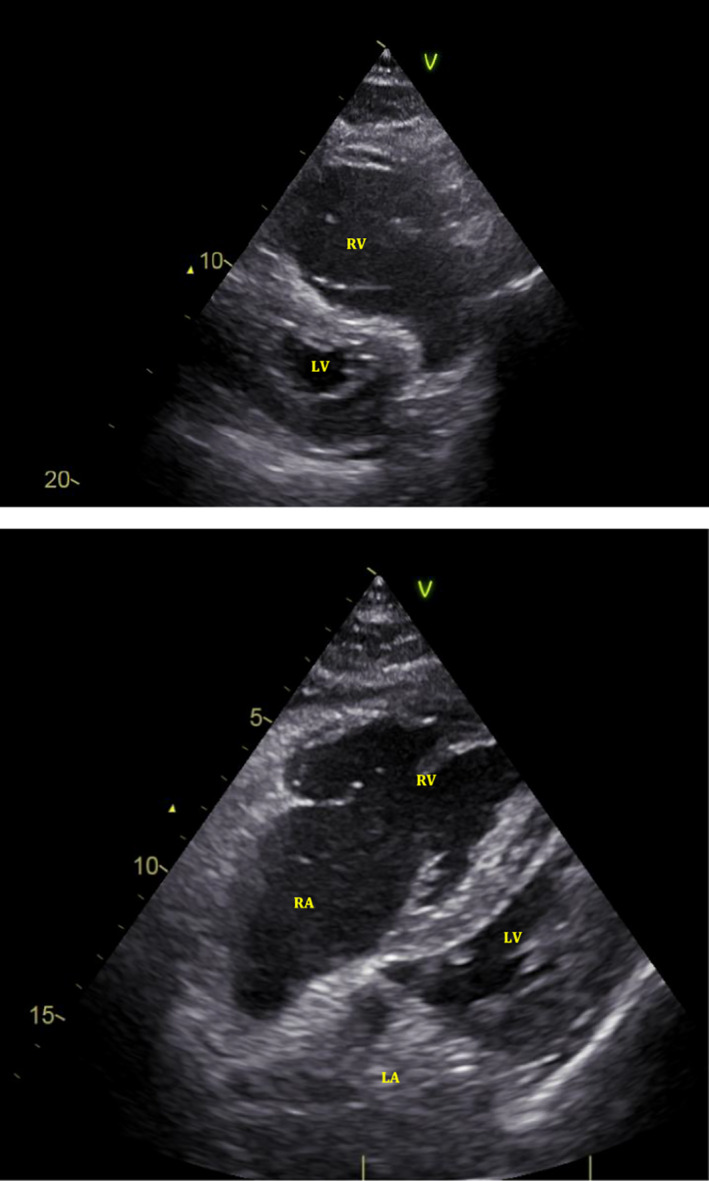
Echocardiography demonstrated massive dilatation of right heart chambers and acute right ventricular failure, with positive McConnell's sign. LA; left atrium, LV; left ventricle, RA; right atrium, and RV; right ventricle
